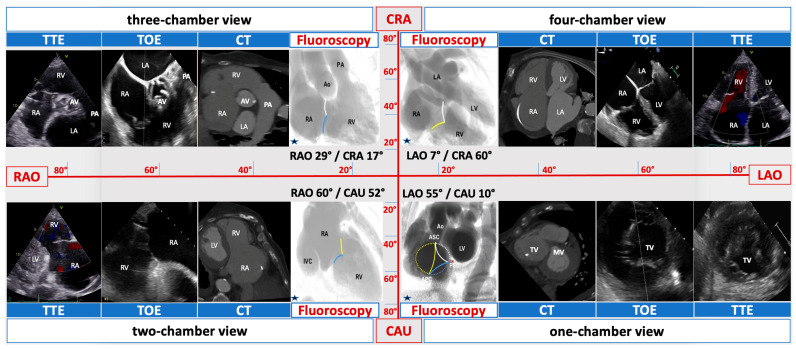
Multimodality imaging of the right heart. Matching echocardiographic transesophageal (TEE) and transthoracic (TTE), CT and fluoroscopic views of right-sided heart structures in a patient scheduled for T-TEER. RV—right ventricle, RA—right atrium, TV—tricuspid valve, MV—mitral valve, AV–aortic valve, PA-pulmonary artery, LV—left ventricle, LA—left atrium, CAU—caudal, CRA—cranial, LAO–left anterior oblique, RAO—right anterior oblique, blue star: reproduction of angiographic images with permission from Elsevier/JACC [13].
